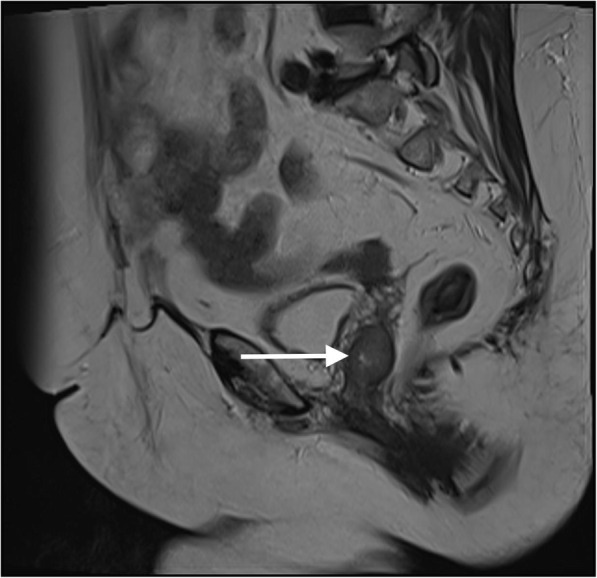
Sagittal T2-weighted image demonstrates lesion (arrow) with intermediate T2 signal intensity filling vaginal lumen
